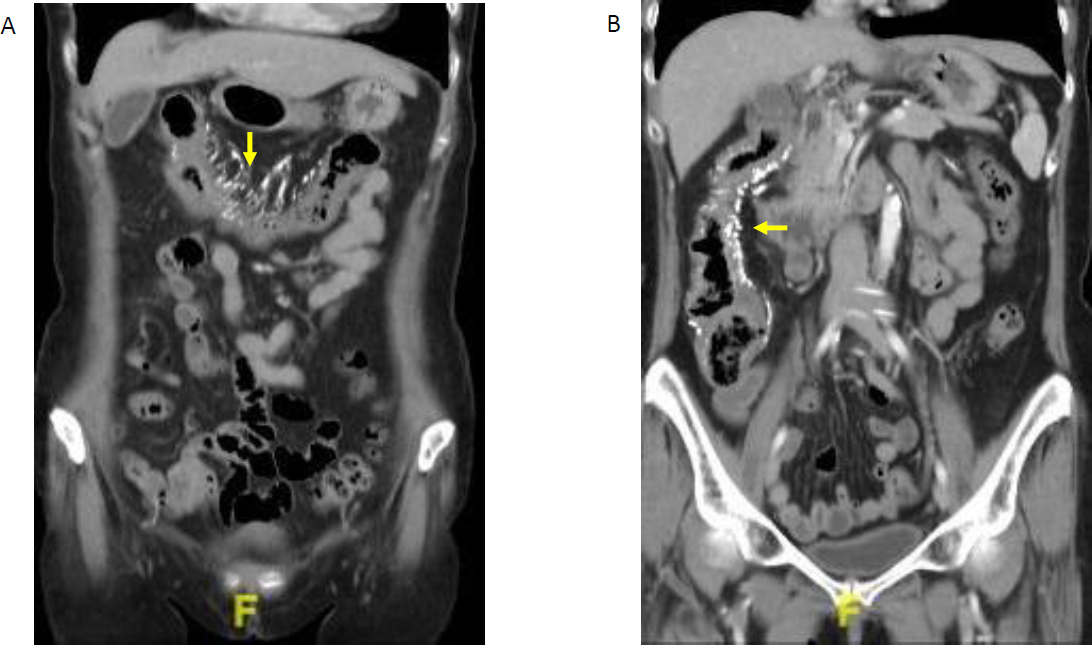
Computed tomography of the abdomen, coronal view, done at a regional hospital, shows characteristic vascular calcification of the pericolic arcades in the distribution of the (A) transverse and (B) ascending colons.
